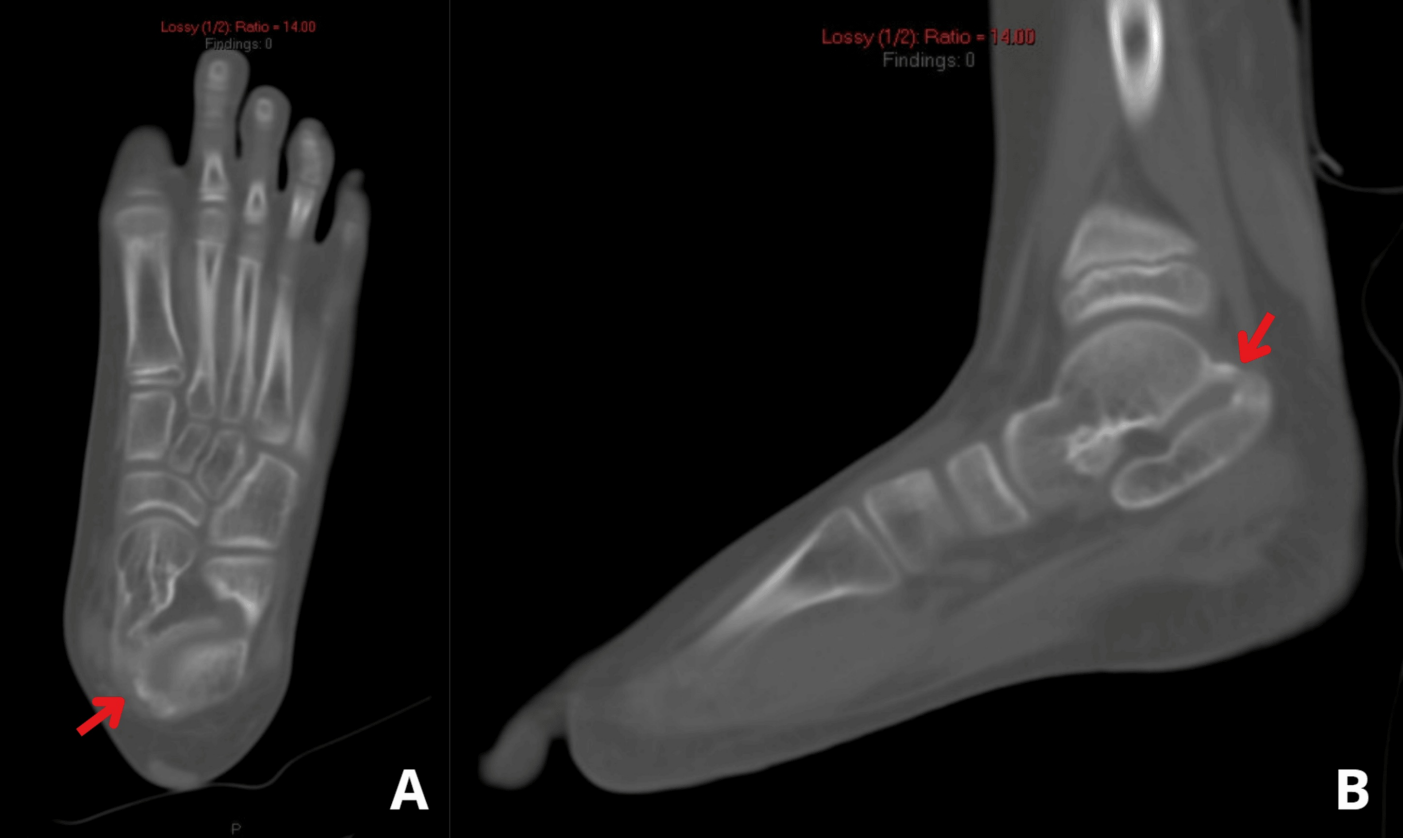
Computed tomography of the left foot demonstrating (A) a transverse view and (B) a sagittal view, revealing an osseous talocalcaneal coalition (red arrow).
